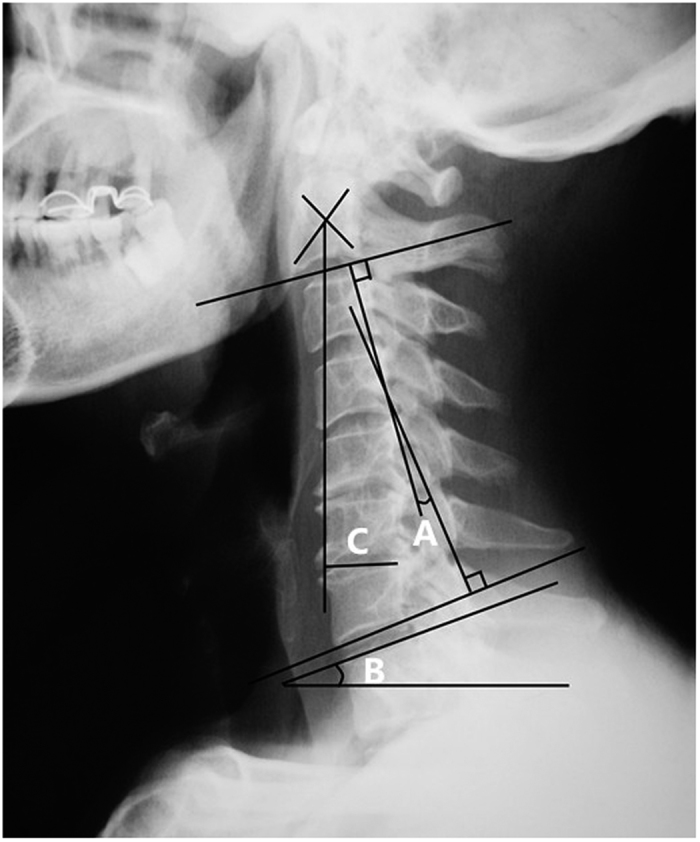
Radiographic measurements. (A) C2–7 Cobb angle. (B) T1 slope. (C) C2–7 SVA. SVA indicates sagittal vertical axis.
